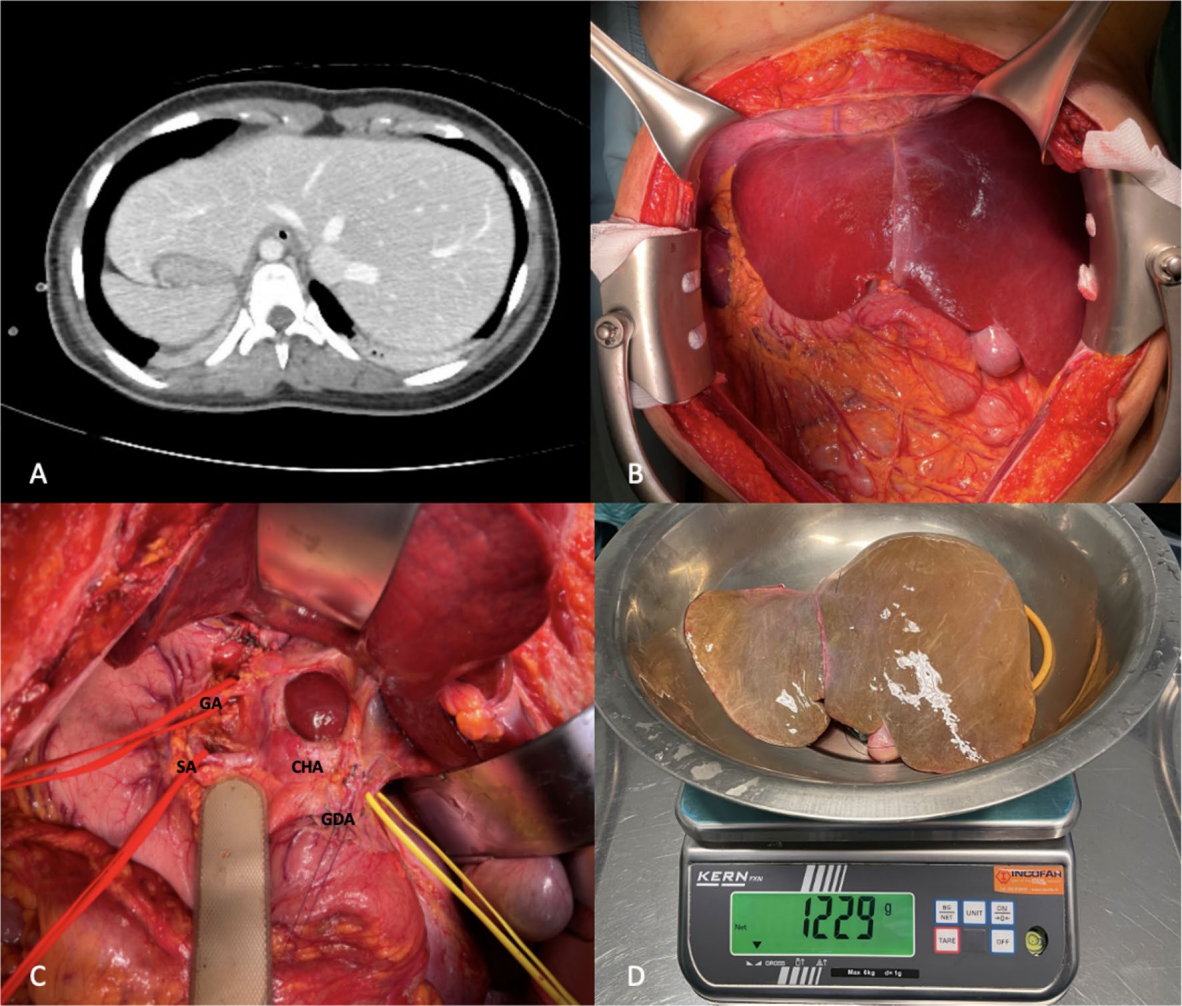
A, CT scan of the donor at the moment of procurement; B, Macroscopic appearance of the liver; C, Preparation of the hepatic hilum, gastric (GA) and splenic artery (SA) on red vessel loops, common biliary duct on yellow vessel loop, gastroduodenal artery (GDA) on violet tie and common hepatic artery (CHA); D, Harvested graft after flush
